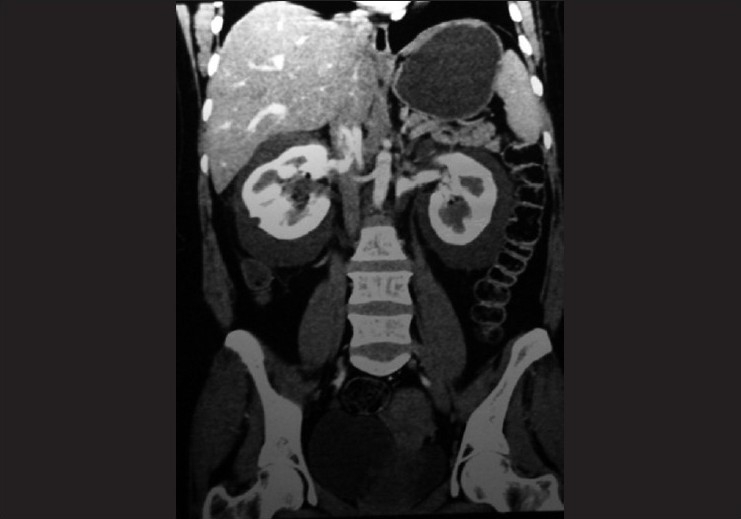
Coronal CT image showing bilateral perinephric collections with otherwise normal kidneys
